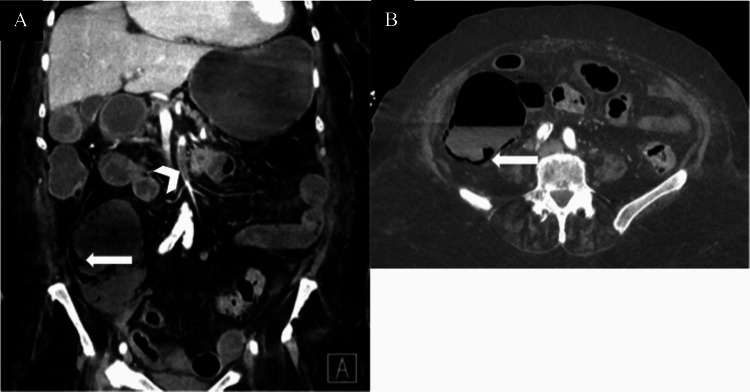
Coronal (A) and axial (B) CT images show superior mesenteric artery (SMA) occlusion (arrowhead) and PI of the colon (arrows). This patient underwent a SMA thrombectomy and resection of 83 cm of ischemic small bowel and 17 cm of ischemic large bowel including the cecum and appendix
